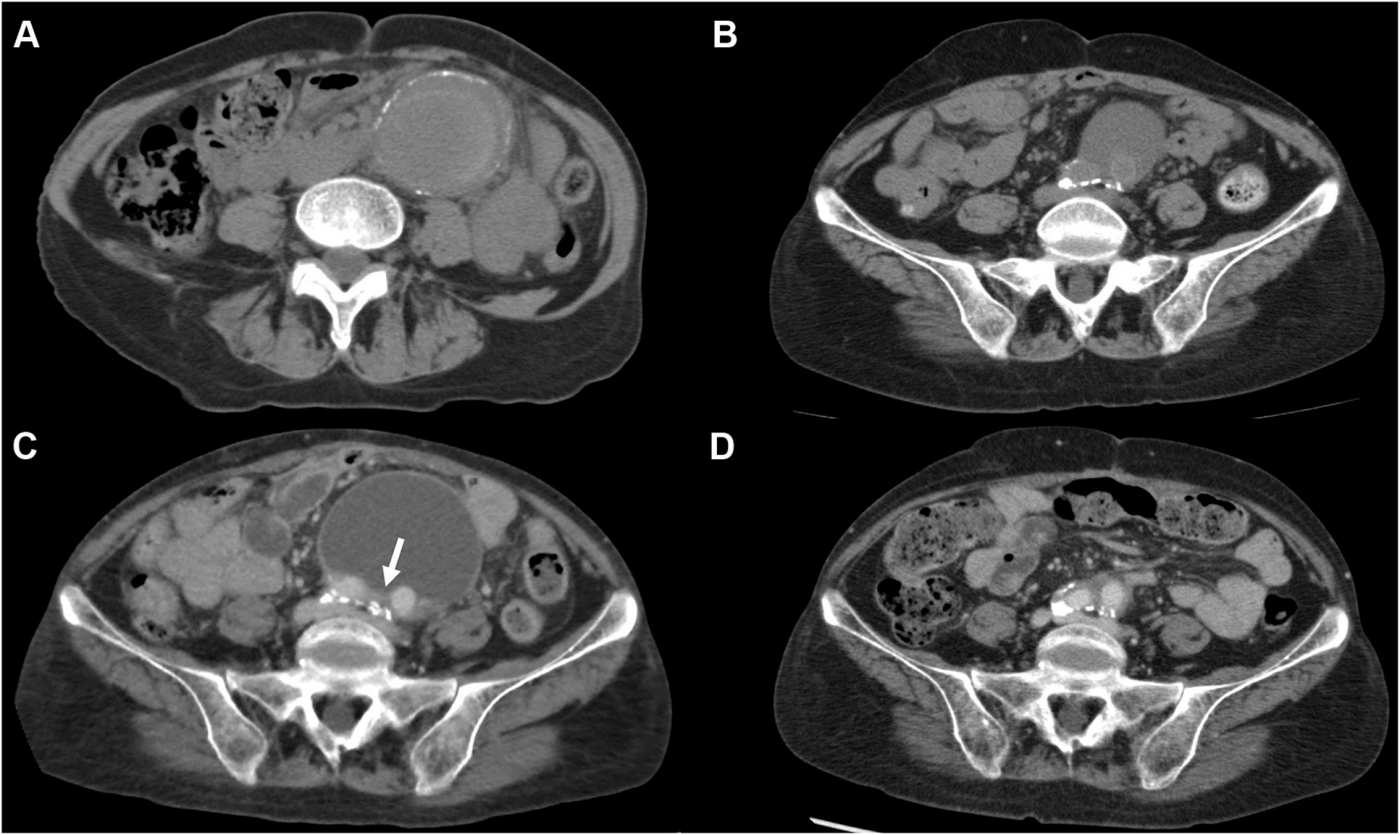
(A) Computed tomography (CT) scans showing an impending rupture of a 54-mm infected abdominal aortic aneurysm (AAA). (B) CT scans showing a 40-mm sac covering the graft 9 years after repair of the aneurysm. (C) Contrast-enhanced CT scans showing an 80-mm sac filled with low-density fluid around the graft. The white arrow indicates the contrast medium flowing into the sac. (D) Contrast-enhanced CT scans showing a small amount of fluid around the graft and no contrast medium flowing into the sac (11 years after repair of the aneurysm and 3 years after laparotomy).
